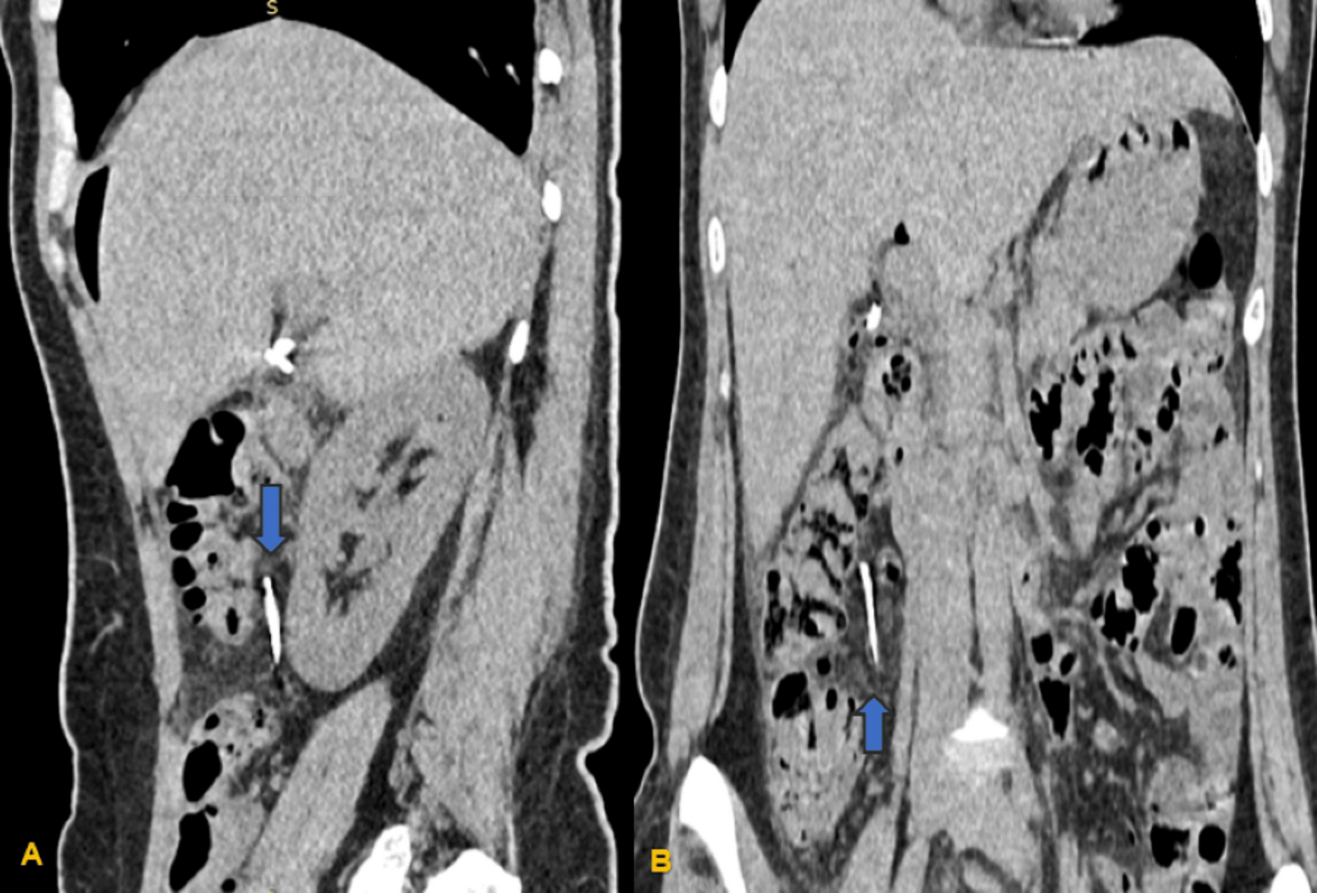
(A) Sagittal plane showing the presence of a longitudinal sharp object (blue arrow) in front of the lower pole of the right kidney located posterior to the ascending colon. (B) Coronal plane of the CT abdomen showing the presence of a sharp foreign body (blue arrow) inferior to the umbilicus, 6-7 cm to the right of the medial line and 3 cm below the right rectus abdominis muscle
